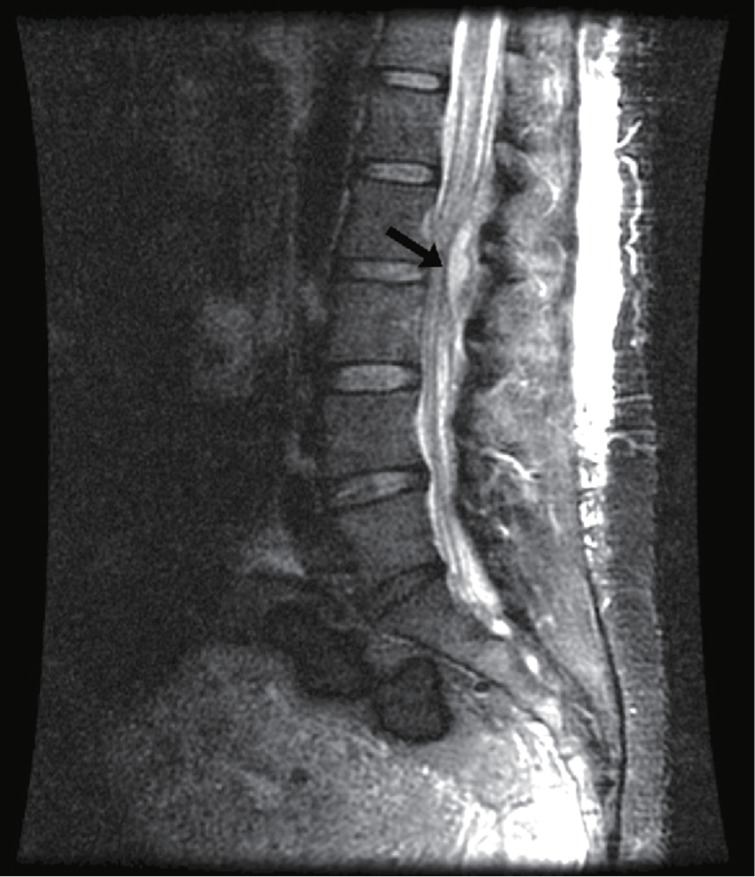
T2 weighted gadolinium MRI image of the lumbar spine. Abnormal enhancement is shows most notably at L2-L3 with 50% cord compression due to a posterior enhancing fluid collection in the epidural space (arrow).
